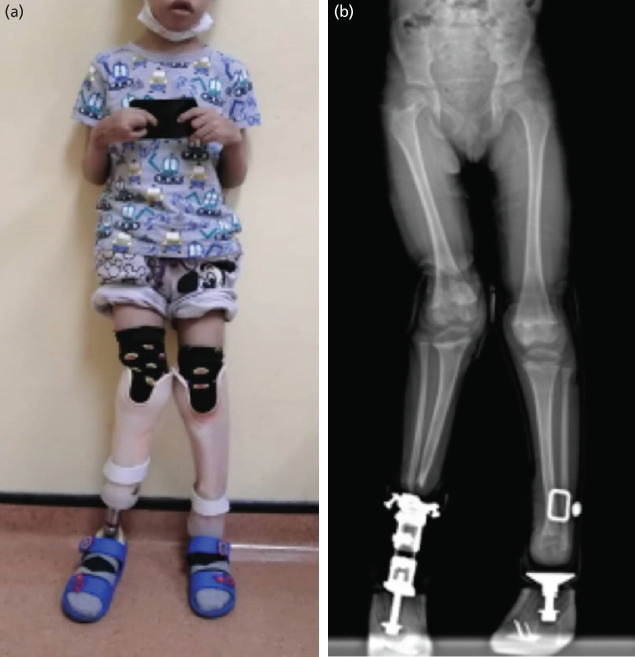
(a) The child at age six years with bilateral below knee prosthesis. (b) The radiograph shows a valgus deformity of the right knee due to asymmetrical growth arrest of the proximal tibia. Physeal irregularities are also seen proximally and distally in the right femur, left distal femur and left distal tibia.
